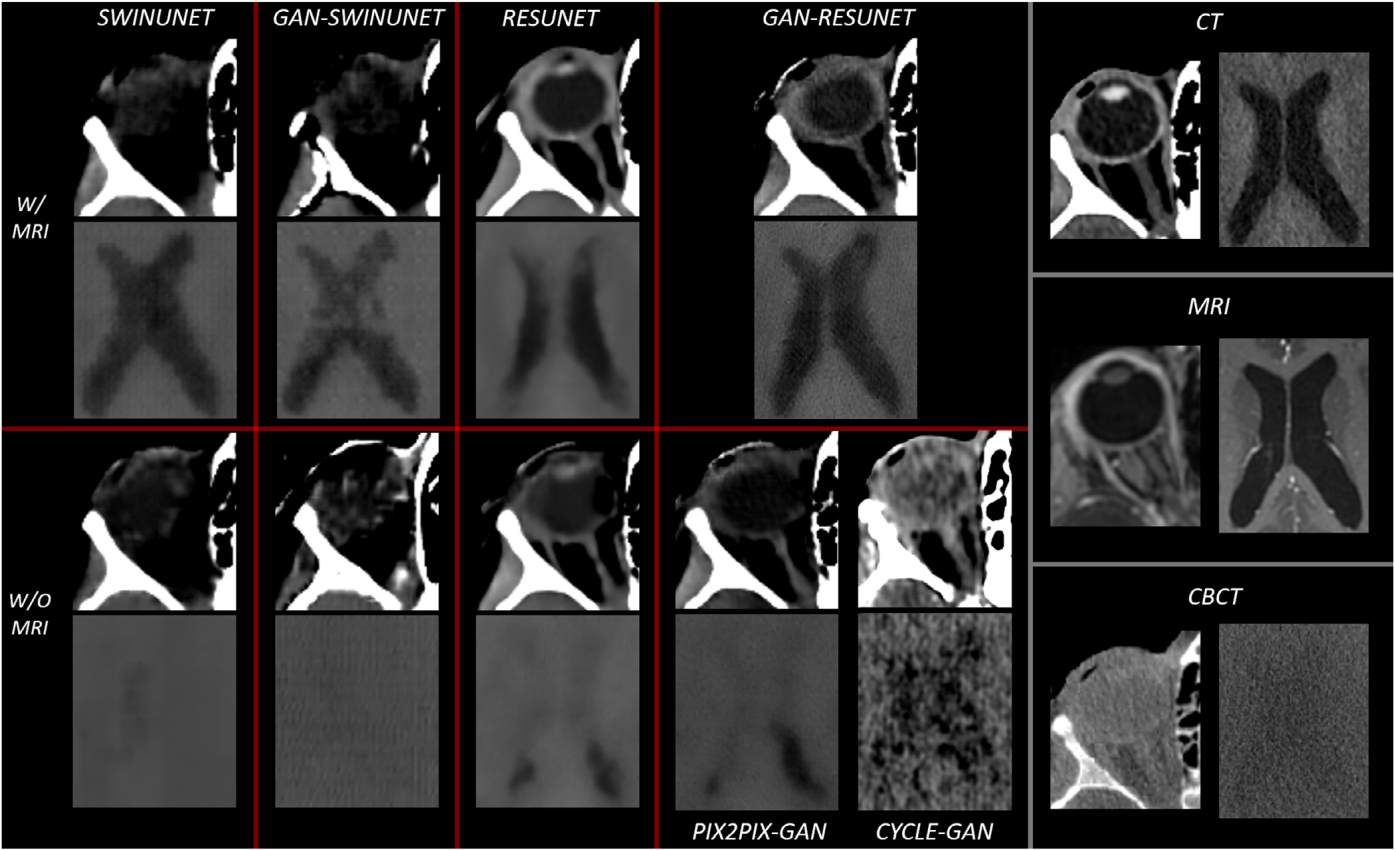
Qualitative visualization for eyes and ventricles in a specific slice. Window width (100 HU) and level (50 HU) are adjusted to facilitate soft tissue visualization. Our nine sCTs related to the different models are presented along with the original CBCT, CT, and MR images. CT, computed tomography; CBCT, cone‐beam CT; HU, Hounsfield units; sCTs, synthetic CTs.
